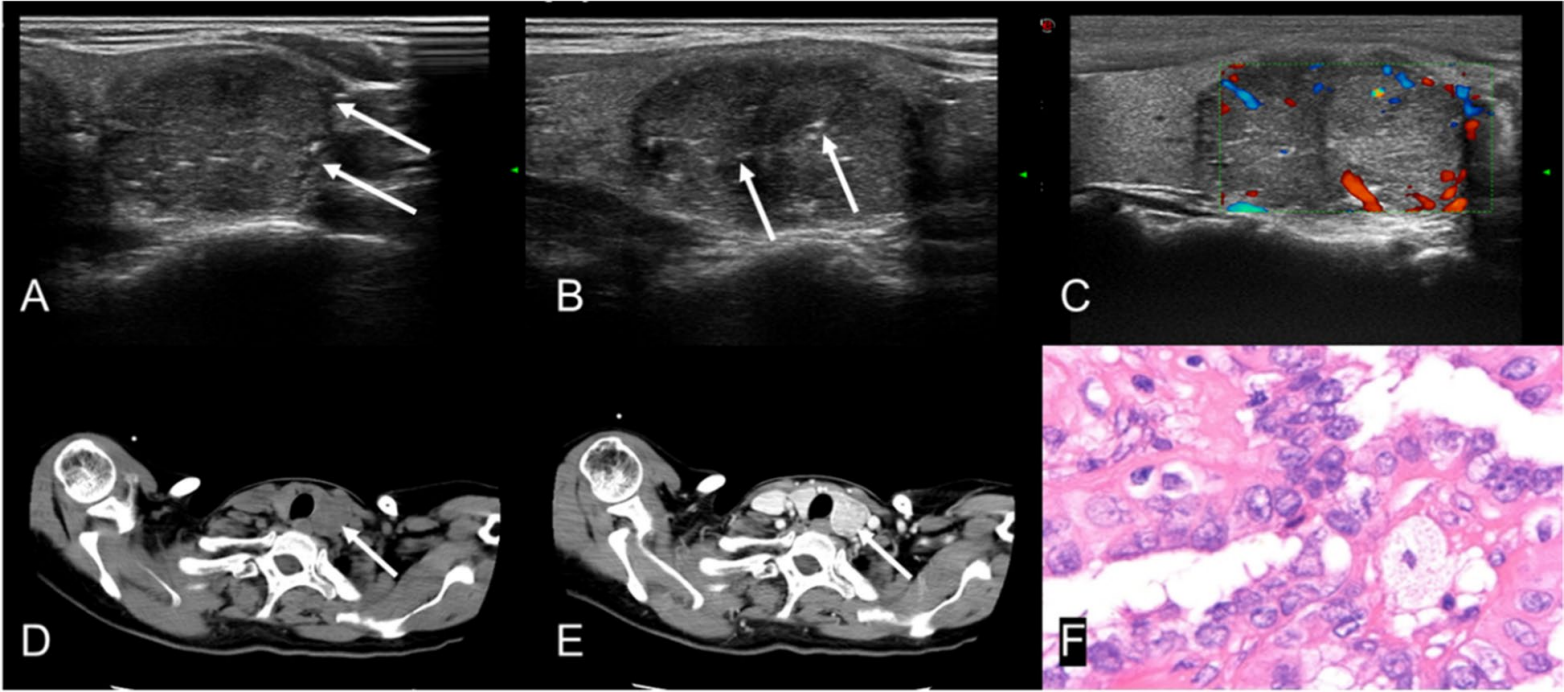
A 50-year-old female patient with neck tumor for 3 months (A) Transverse image of ultrasonography, showing heterogeneous hypoechoic nodules on the left lobe of thyroid with irregular border; (B) Longitudinal image of ultrasonography. The arrows show the internal small calcification; (C) Blood flow spectrum, showing rich blood flow signals; (D) Non-contrast CT, showing low-density nodules on the left lobe of thyroid; (E) Venous phase CT, showing heterogeneous significant enhancement of nodules in the left lobe of thyroid; (F) Pathological results, showing papillary thyroid carcinoma (* 400)
